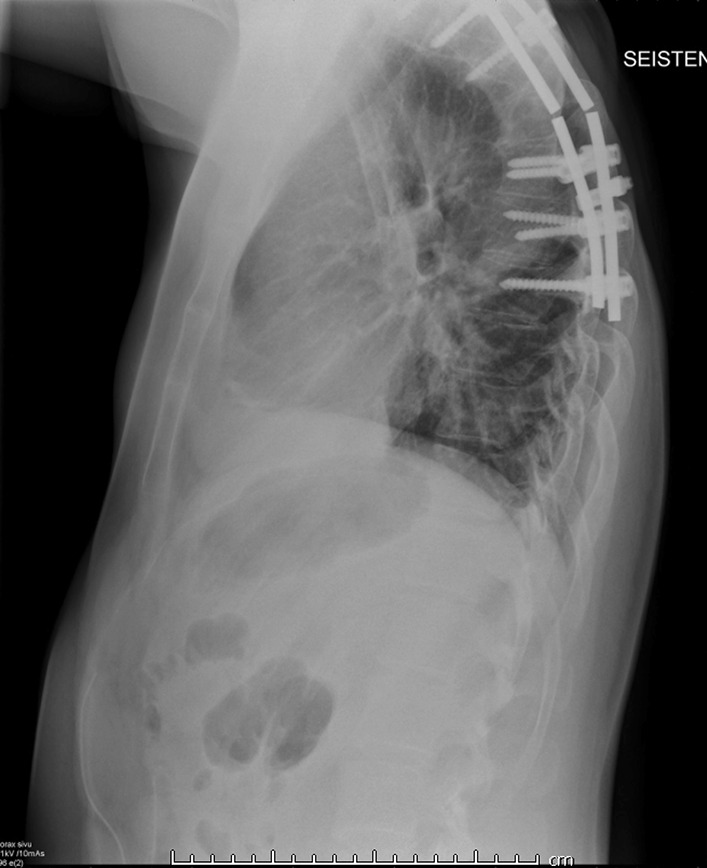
Destruction of the fixation materials on chest x-ray
